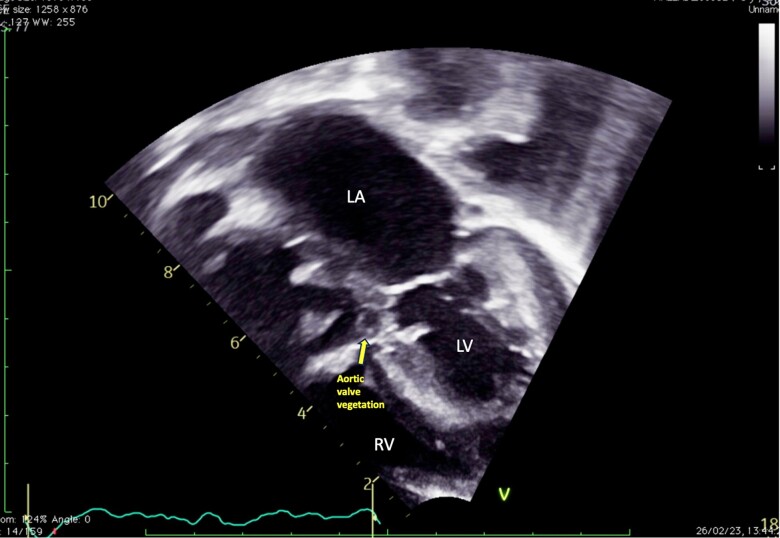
Transthoracic echocardiography performed at admission. Five-chamber view showing aortic valve vegetation.
